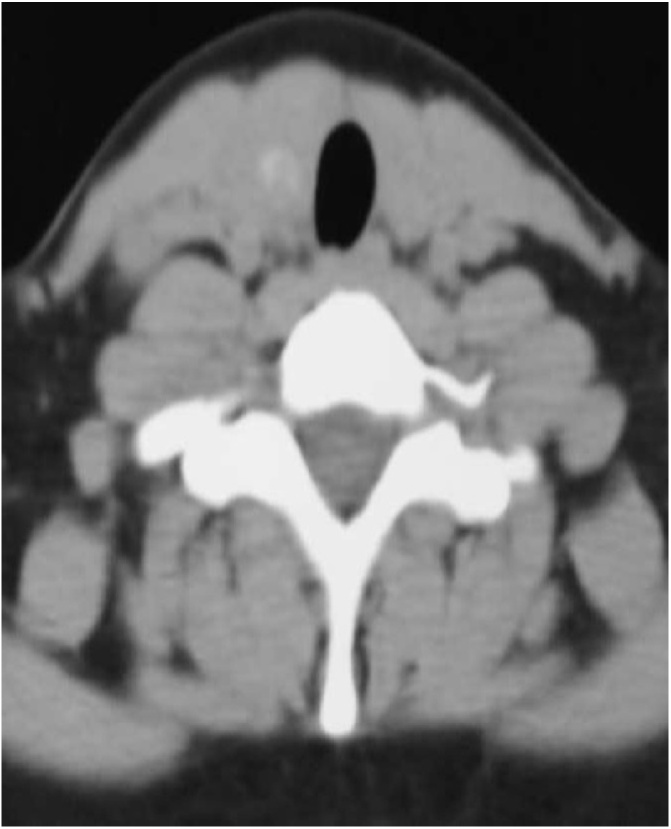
A 16 year old girl with bilateral papillary thyroid carcinoma. (A) Axial enhanced CT neck showing enhancing mass lesion involving right thyroid lobe. The left lobe appears to be normal in CT scan.
